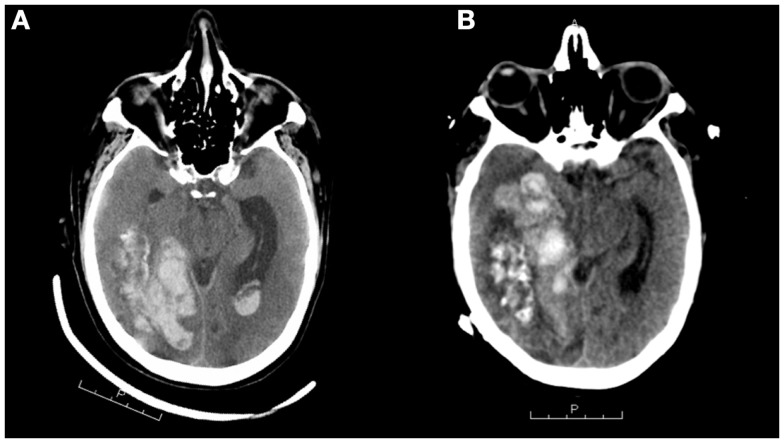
CT scans (A,B) demonstrating intracranial hemorrhage 3 years after initial SAH. The left scan shows a large right temporal hematoma that was emergently evacuated via craniotomy. The right scan, taken 1 month later, shows extensive intraparenchymal hemorrhage centered in the right temporal lobe extending into the right parietal and occipital lobes measuring up to 7.8 cm × 3.7 cm. There is bleeding into the ventricular system. Note the mass effect, causing midline shift toward the left of 7 mm and uncal herniation.
